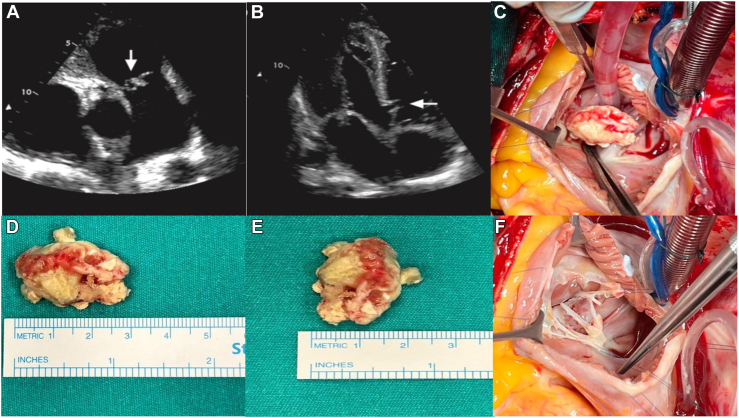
Preoperative and intraoperative findings of tricuspid valve endocarditis and ventricular septal defect repair. (A) Preoperative echocardiogram showing vegetation on the tricuspid valve (arrow). (B) Preoperative echocardiogram showing the ventricular septal defect (arrow). (C) Intraoperative view of the vegetation on the tricuspid valve. (D, E) Vegetation after surgical resection. (F) Tricuspid anterior cusp after resection of the vegetation, showing ruptured chordae.
